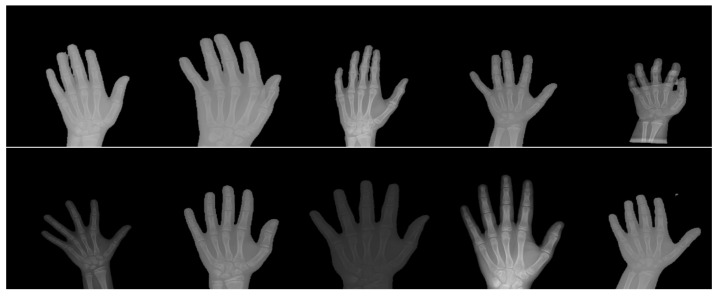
Some samples of the X-ray image that have been normalized by the three normalization modules.
